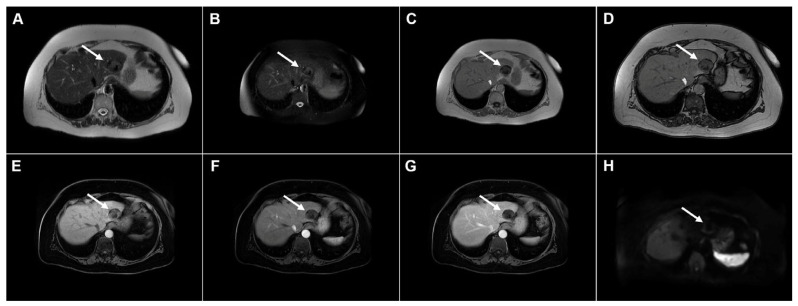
Cystic echinococcosis, WHO stage CE5, in a 72-year-old man. Axial T2-weighted (A) and T2-weighted fat-suppressed (B) images demonstrate a heterogeneous, predominantly hypointense, solid-appearing lesion (*arrow*) in liver segment II. In-phase (C) and out-of-phase (D) images show the lesion (*arrow*) as hypointense, with a signal void along the cyst wall consistent with calcifications. In the T1-weighted fat-suppressed image (E), the lesion is heterogeneously hypointense, with a linear hypointense rim along the cyst wall (*arrow*), compatible with calcifications. No enhancement can be observed in the arterial (F) or portovenous phase (G) (*arrow*). In diffusion-weighted imaging (H), the lesion is isointense relative to the surrounding liver parenchyma (*arrow*).
